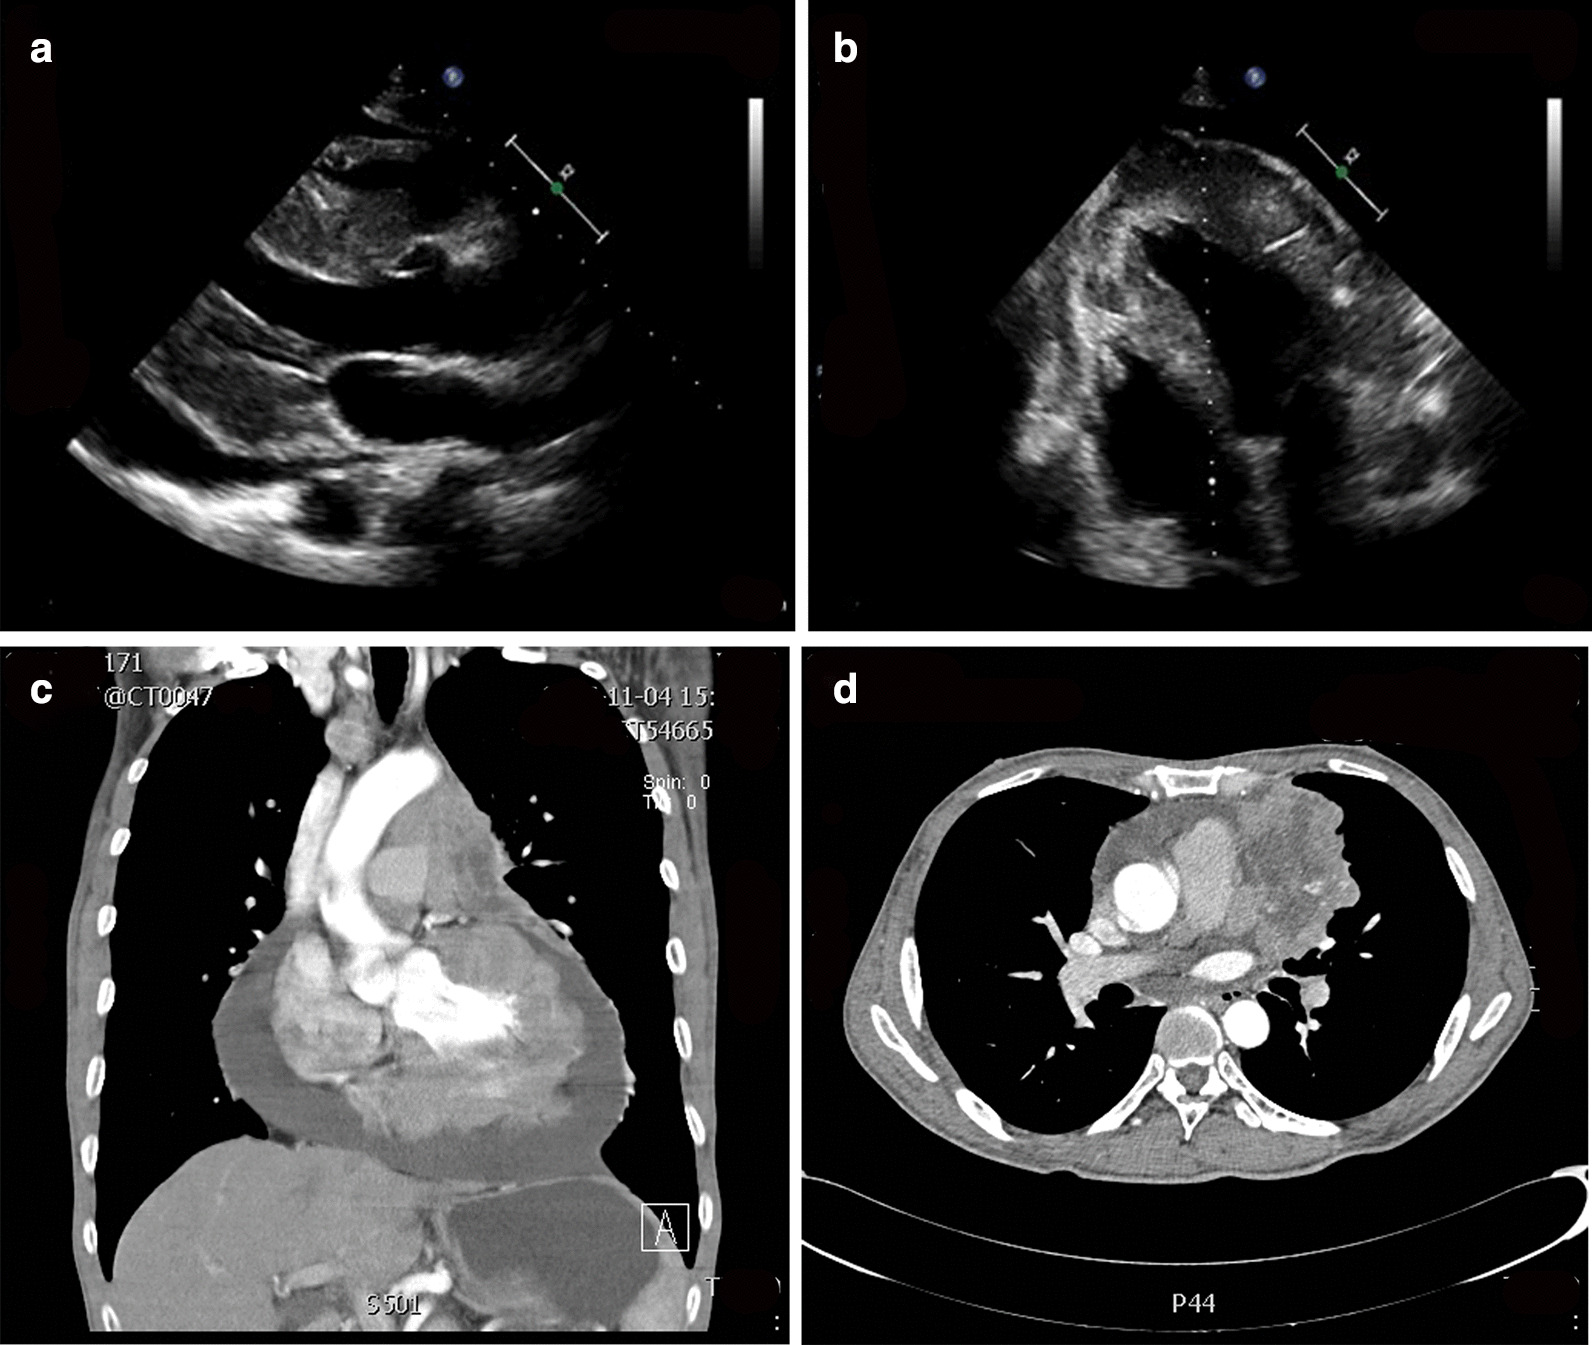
Echocardiography and chest CT scans. a, b Echocardiography showed severe pericardium effusion, thickened visceral pericardium, asymmetric septal hypertrophy, and thickened and nonhomogeneous ventricular walls with irregular echo-density of the myocardium. c, d CT scans showed an irregularly shaped mass (6.0 cm × 6.9 cm) in the anterior mediastinal region that had invaded the pericardium and left ventricular walls
